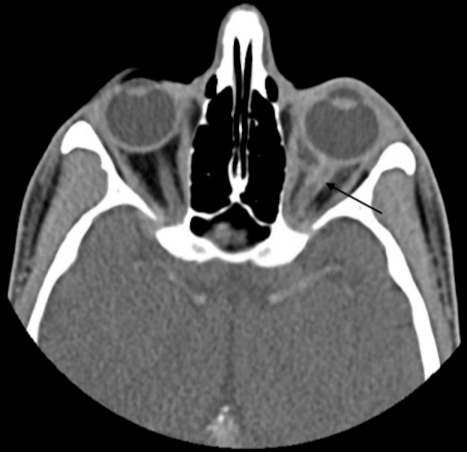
Computed tomography showing a retrobulbar orbital mass in the left orbit (black arrow)
